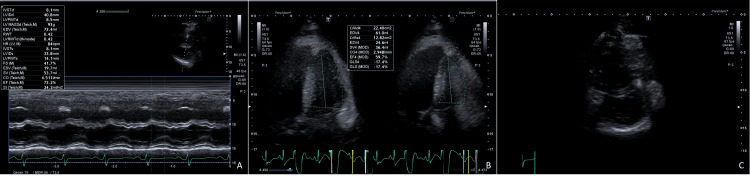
Echocardiographic ventricular function and interdependence. (A) Preserved shortening fraction, concentric remodeling of the left ventricle, and diastolic displacement of the septal wall to the left. (B) Ejection fraction by the biplane disc method (Simpson) in four chambers normal (59%). (C) Ventricular interdependence with diastolic displacement of the septum to the left (volume overload).
